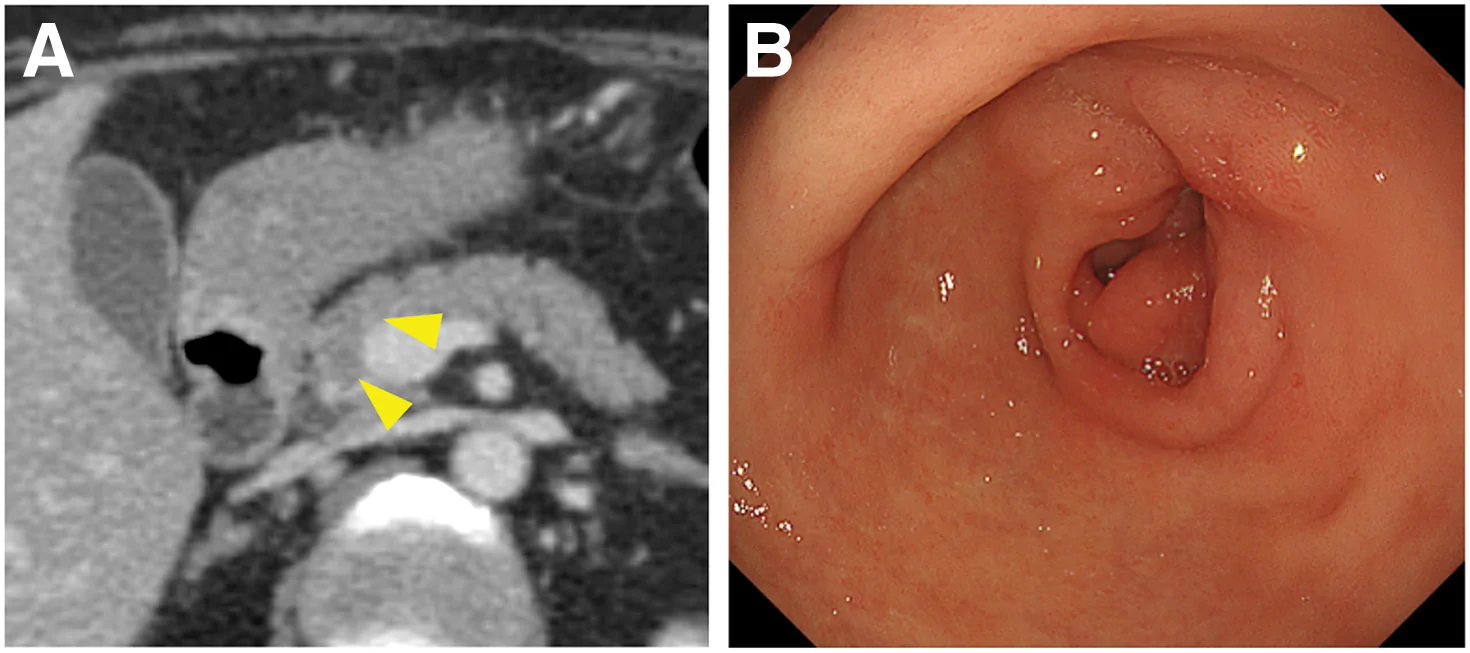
Response to chemotherapy. (A) Contrast-enhanced CT after chemotherapy demonstrating marked regression of the pancreatic invasion (arrowheads). (B) Upper gastrointestinal endoscopy after chemotherapy showing marked regression of the type 4 lesion, with improved luminal distensibility and residual ulcerative changes.
